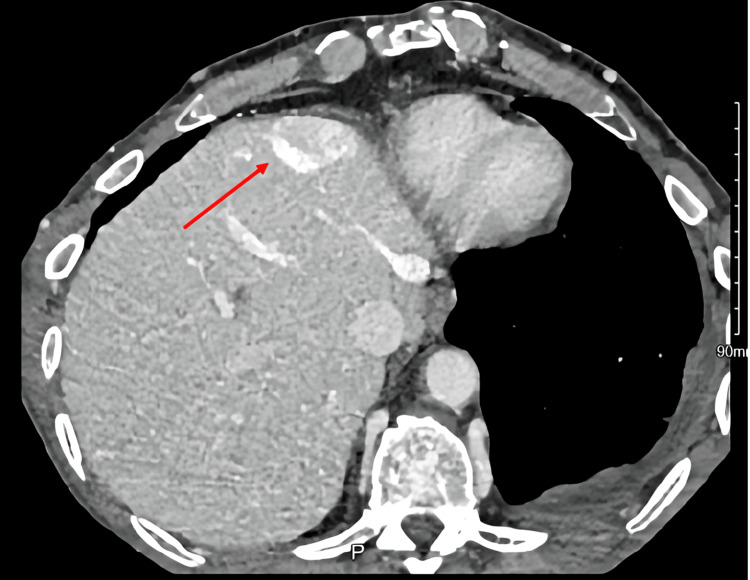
Axial view of CT abdomen showing hot quadrant sign in segment IV of liver
